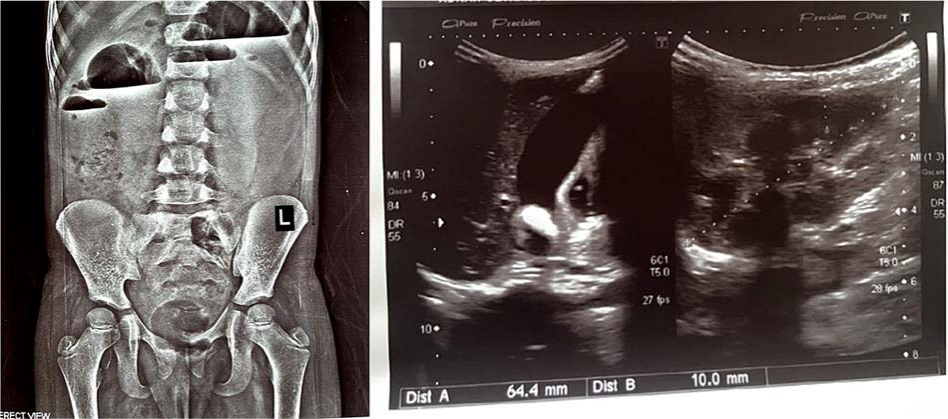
X-ray abdomen erect showing air fluid levels (left). Ultrasound abdomen showing intussusception (right).
